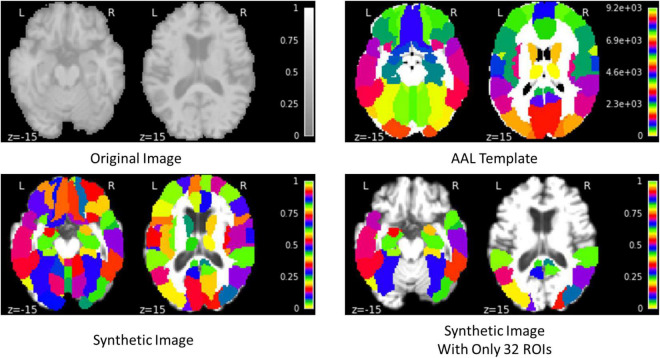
Example of generating the synthetic MRI image.
